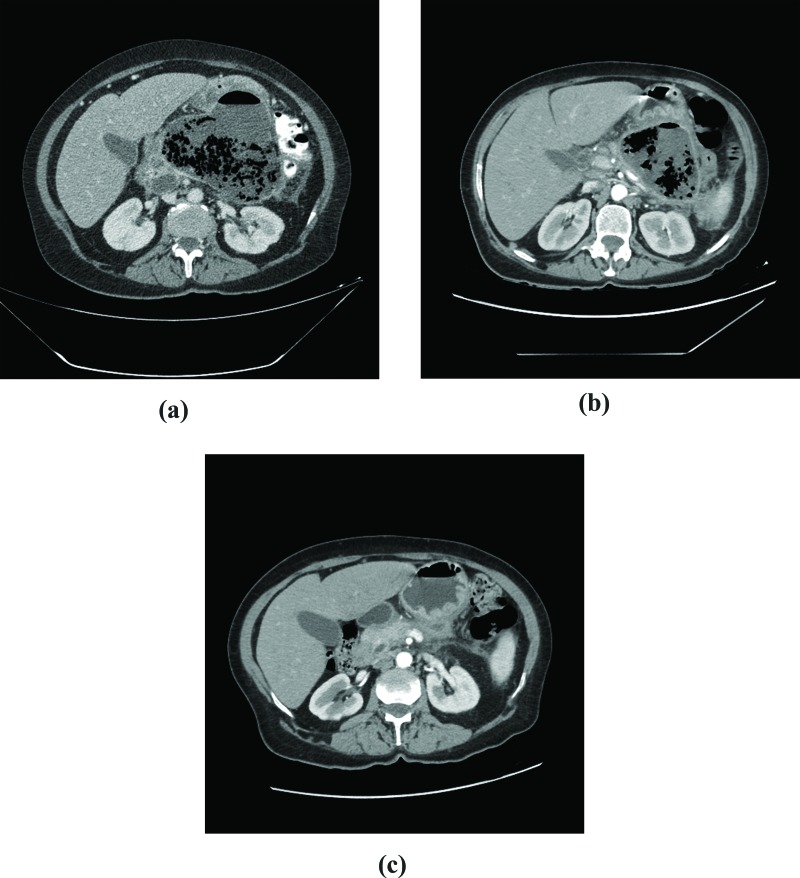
CT scan, case 4. A: First exam, 3 weeks after the onset of symptoms: extensive pancreatic necrosis with a large amount of gas in the body and tail of the pancreas. B: After 60 days of follow-up: a small decrease in the collection of gas is noted. C: 4 months after hospital discharge: atrophy of pancreatic parenchyma.
